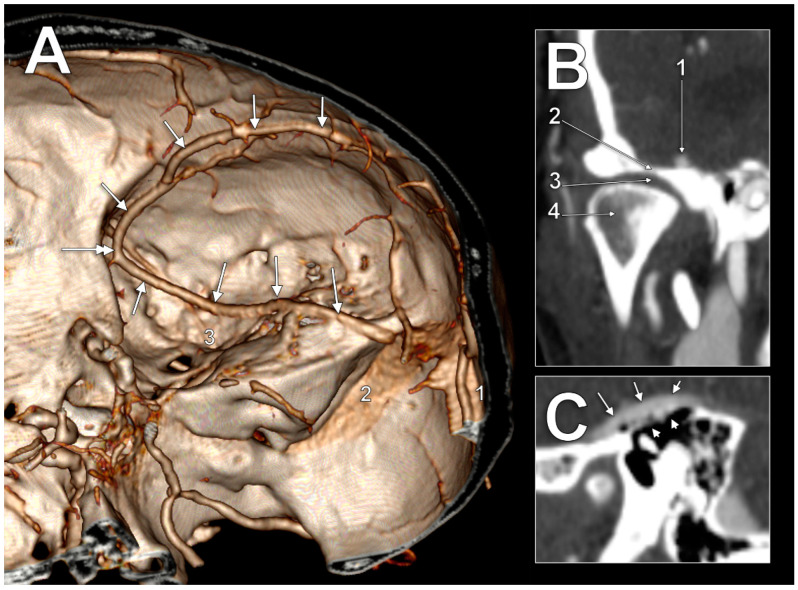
(A) CT angiography, three-dimensional volume rendering of the right superficial middle cerebral vein (SMCV) of the basal type (arrows). Superomedial view. 1. transverse sinus; 2. sigmoid sinus; 3. foramen spinosum. The sagittal loop of the SMCV is indicated (double-headed arrow). (B) Coronal CT slice through the right temporomandibular joint. 1. superficial middle cerebral vein; 2. mandibular fossa; 3. articular disc; 4. mandibular condyle. (C) Sagittal CT slice through the right tympanic cavity. The superficial middle cerebral vein (arrows) courses on a dehiscent tympanic roof (arrowheads).
